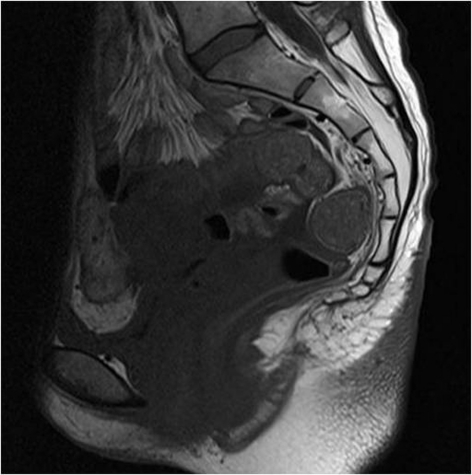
MR image taken 2 years after operation shows no local recurrence.
